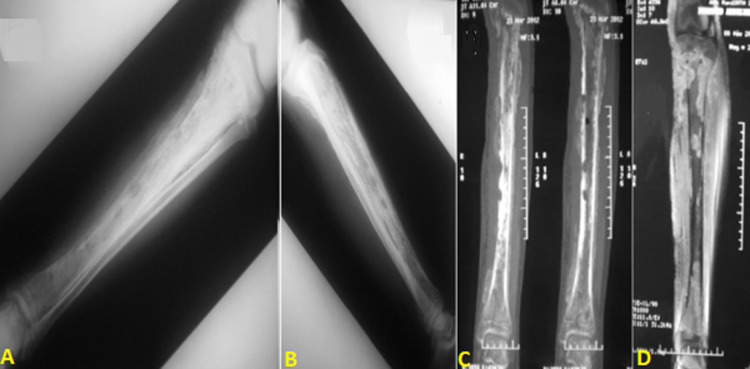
radiographie standard de la jambe (A,B) complétée par une tomodensitométrie (C) et une IRM (D) pour une ostéomyélite de l´extrémité supérieure du tibia compliquée d´une pandiaphysite
